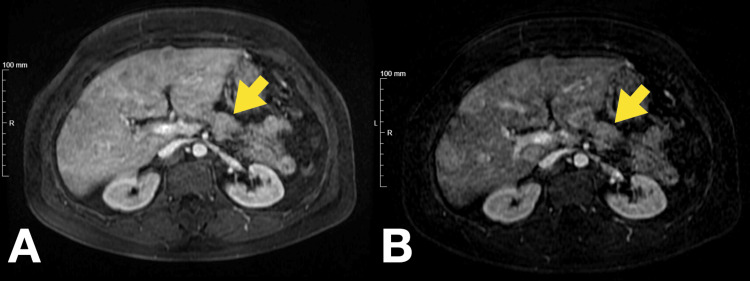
Abdominal MRI showing multiple hypervascular nodules compatible with liver metastasis and hypervascular nodule in the pancreatic body Arterial phase T1 fat-suppressed images showing multiple hypervascular enhancing nodules on the liver consistent with metastatic lesions and a solid enhancing nodule in the pancreatic body, as the primary lesion, compatible with NET MRI: magnetic resonance imaging, NET: neuroendocrine tumor
